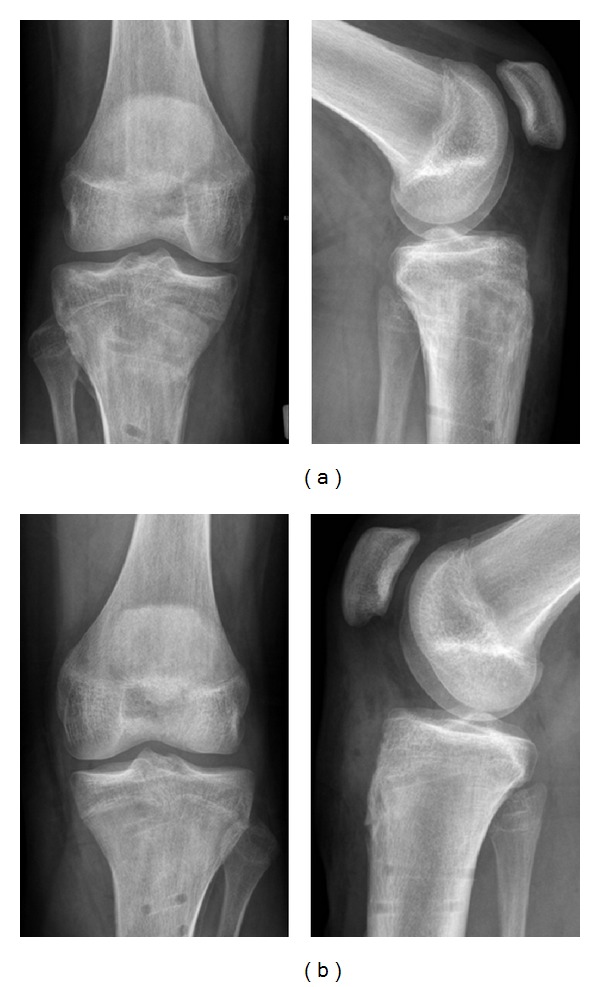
Plain radiographies of the right (a) and left knee (b) following implant removal four months after trauma. Bony healing in anatomic position, growth plates fused.
